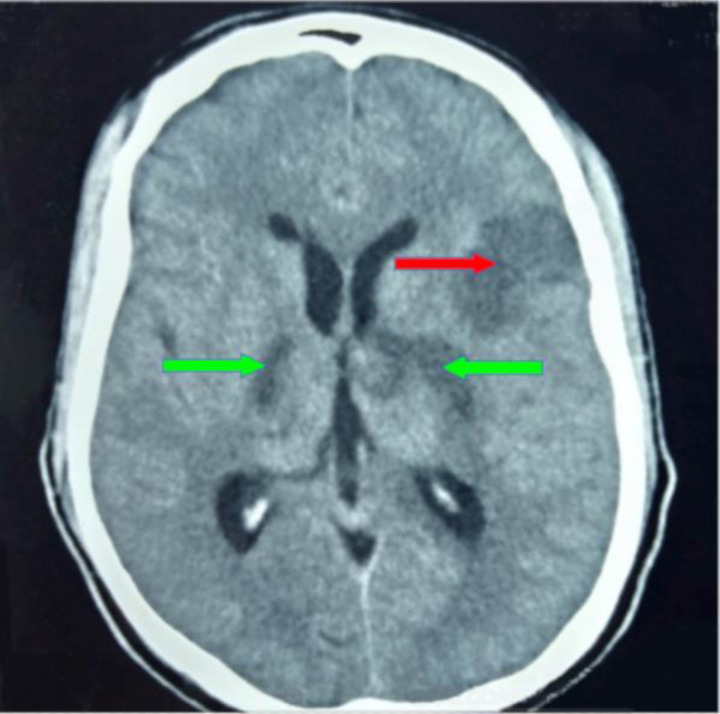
An axial non-enhanced CT scan of the brain showing an extensive area of hypodensity in the region of the left temporoparietal brain with associated sulcal flattening/effacement (wedge-shaped hypodensity extending to the edge of the brain) shown with red arrow, and at the basal ganglia areas (significant deep brain hypodensities) worse on the left shown with green arrows, in keeping with acute bilateral basal ganglia and left temporoparietal infarcts.
